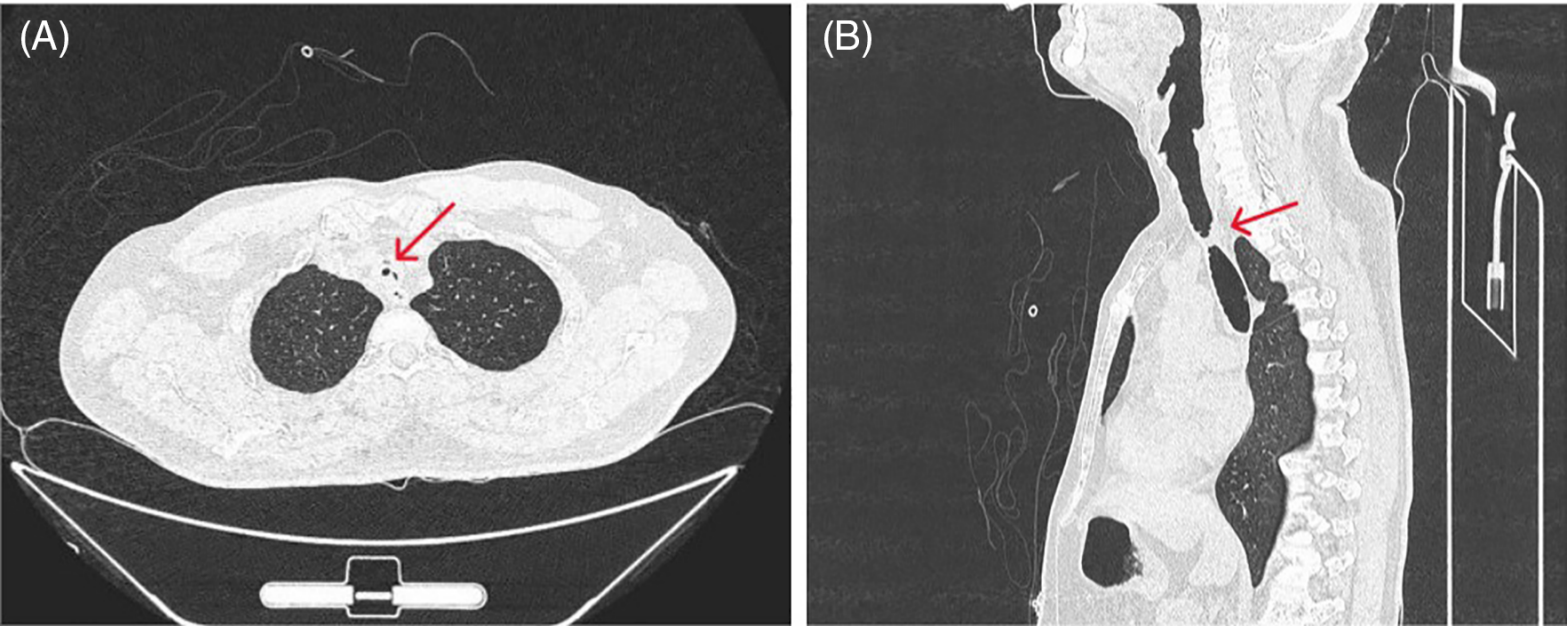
Computed tomography scan of patient‐5 showing complex tracheal stenosis (red arrow) in (A) axial view and (B) sagittal view.
